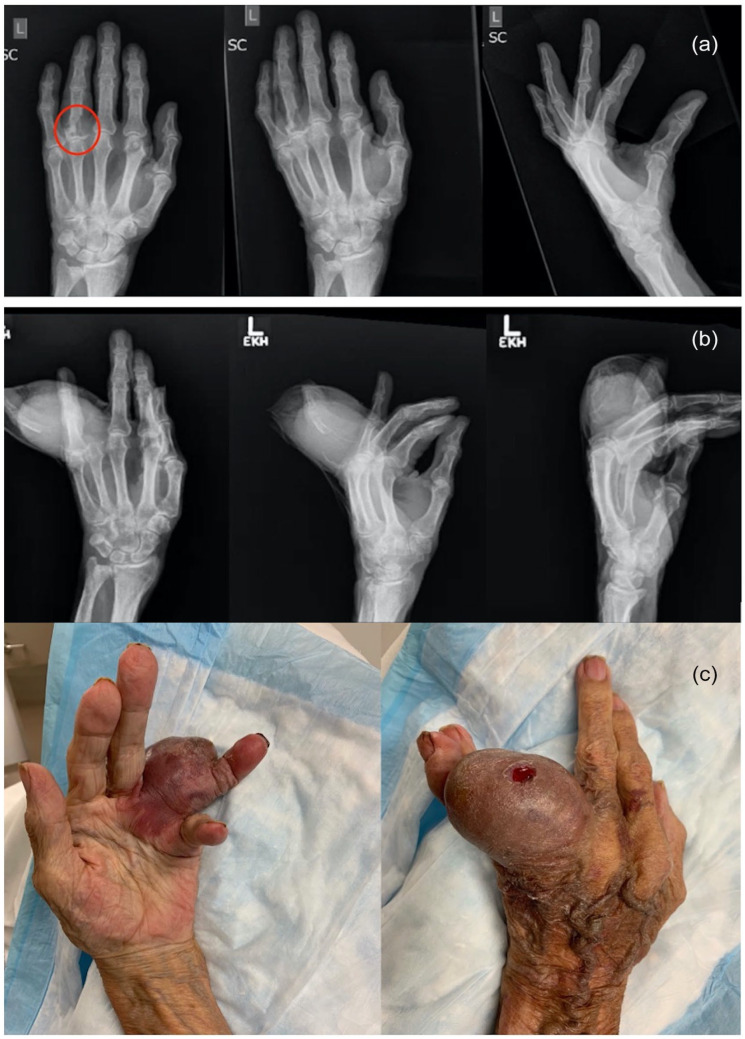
A 78-year-old man with metastatic renal cell carcinoma to the left fourth digit proximal phalanx. *Note*. (a) X-ray imaging at the time of presentation, incorrect diagnosis of gout. (b) X-ray imaging at the time of referral to hand surgery service 7 months later. (c) Clinical appearance of metastatic renal cell carcinoma.
